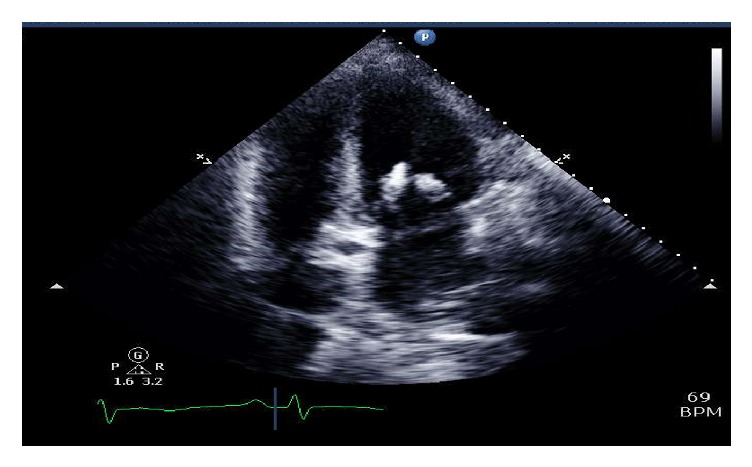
Image depicting a transthoracic echo cardiogram, depicting vegetation and severe mitral regurgitation.
